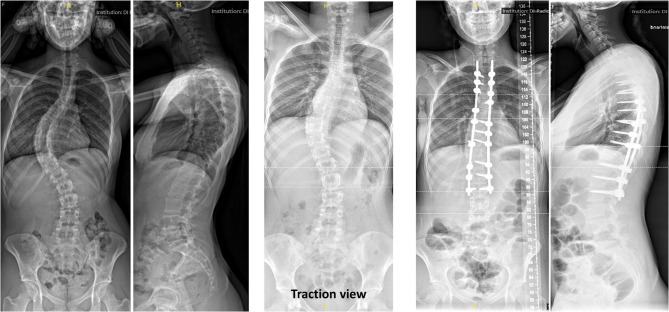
Pre- and postoperative A.P. and lateral radiographs of a 16-year-old female patient with idiopathic adolescent scoliosis, treated with posterior spinal fusion using pedicle screws only
